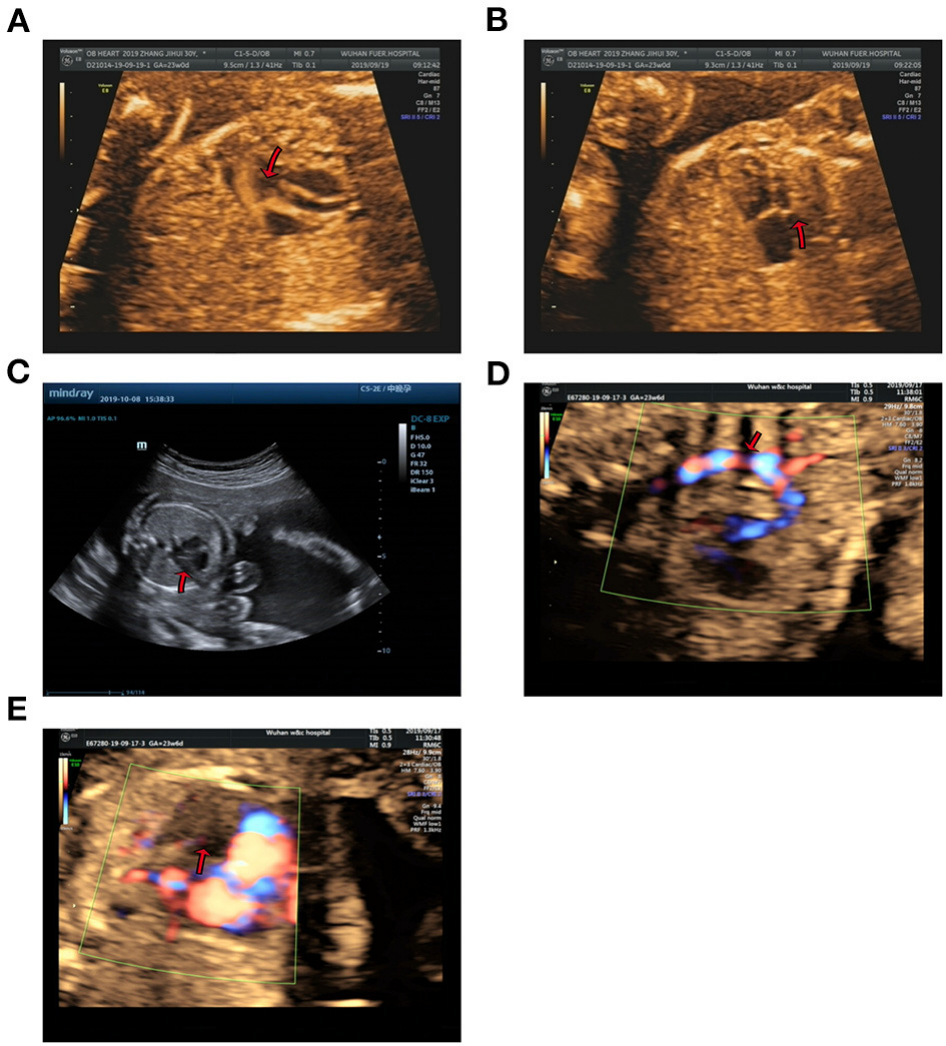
Echocardiographic images of fetal heart structures. (A,B) show fetal ventricular septal defect and hypoplasia of left heart in case 1. (C) displays a smaller left heart in case 2. (D,E) show coarctation of the aorta and mitral atresia in the fetal heart of case 3.
